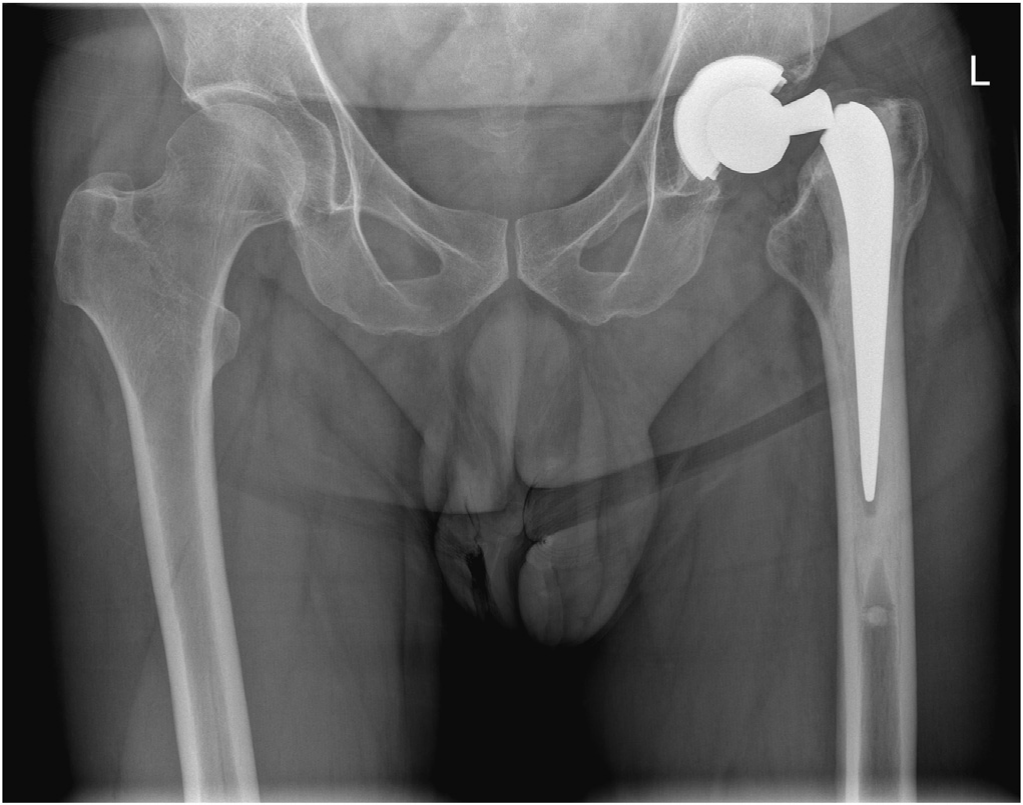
Anteroposterior pelvic plain radiograph showing the neck fracture of the cemented Exeter stem.
